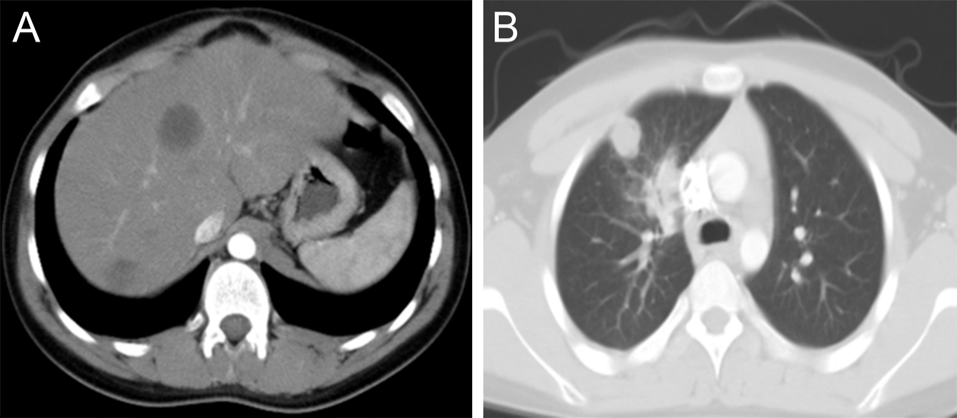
Computed tomography scans of the same patient show hydatid cyst in the right lung upper lobe anterior segment (A) and liver right lobe (B).
